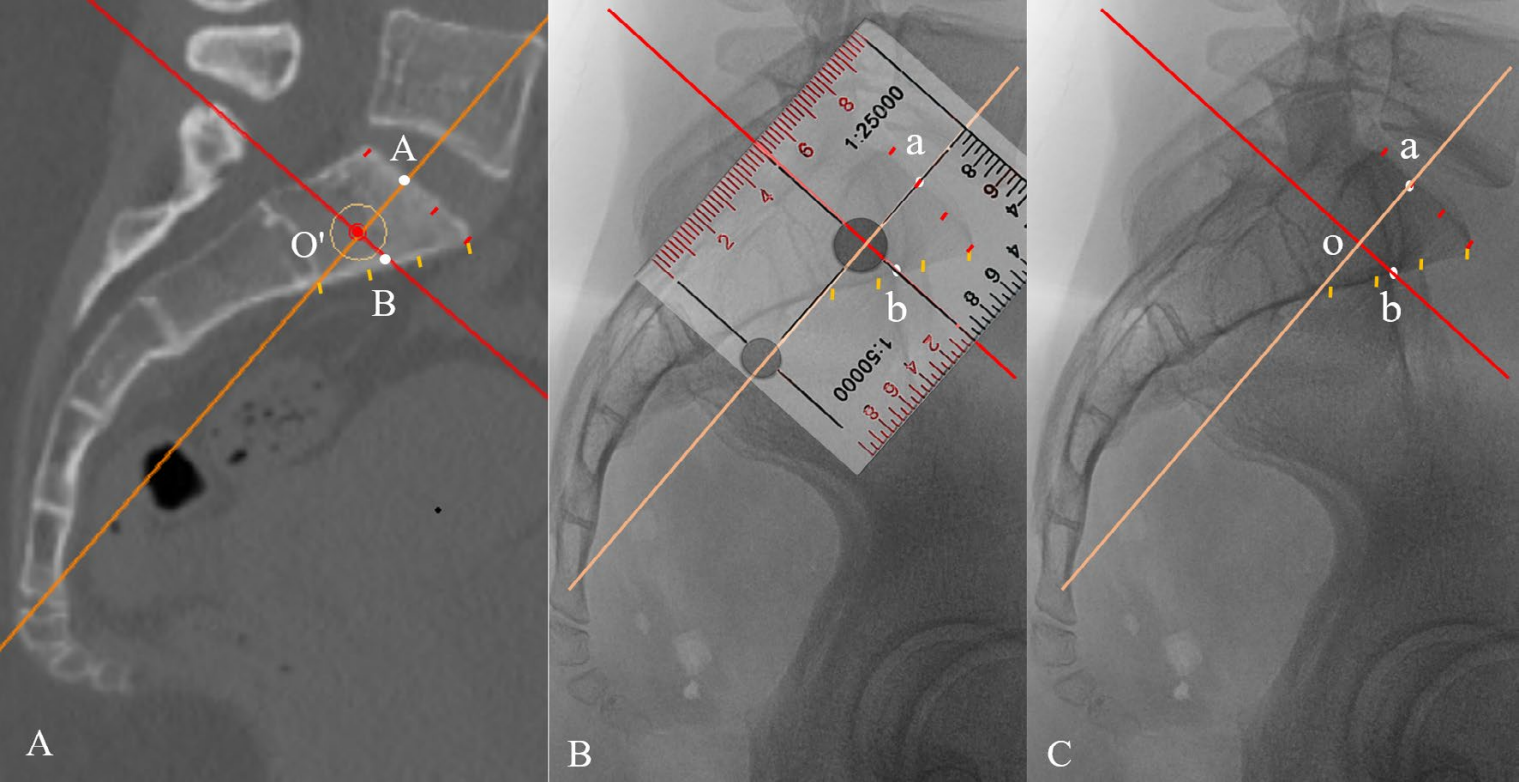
The optimal entry point was formulated in the strict mid‐sagittal plane using Mimics software (A) The red line (axial plane) was parallel to the upper endplate of S1 and intersected the anterior cortex of S1 body (point B). The orange line (coronal plane) was perpendicular to the red line and intersected the superior cortex of S1 body (point A). Point a and b were marked on the pelvic lateral radiograph corresponding to the position A and B. Two lines passing through point a and b were introduced with a coordinate ruler (B). The vertical crossing point o was verified as the optimal entry point for a transiliac‐transsacral screw (C).
